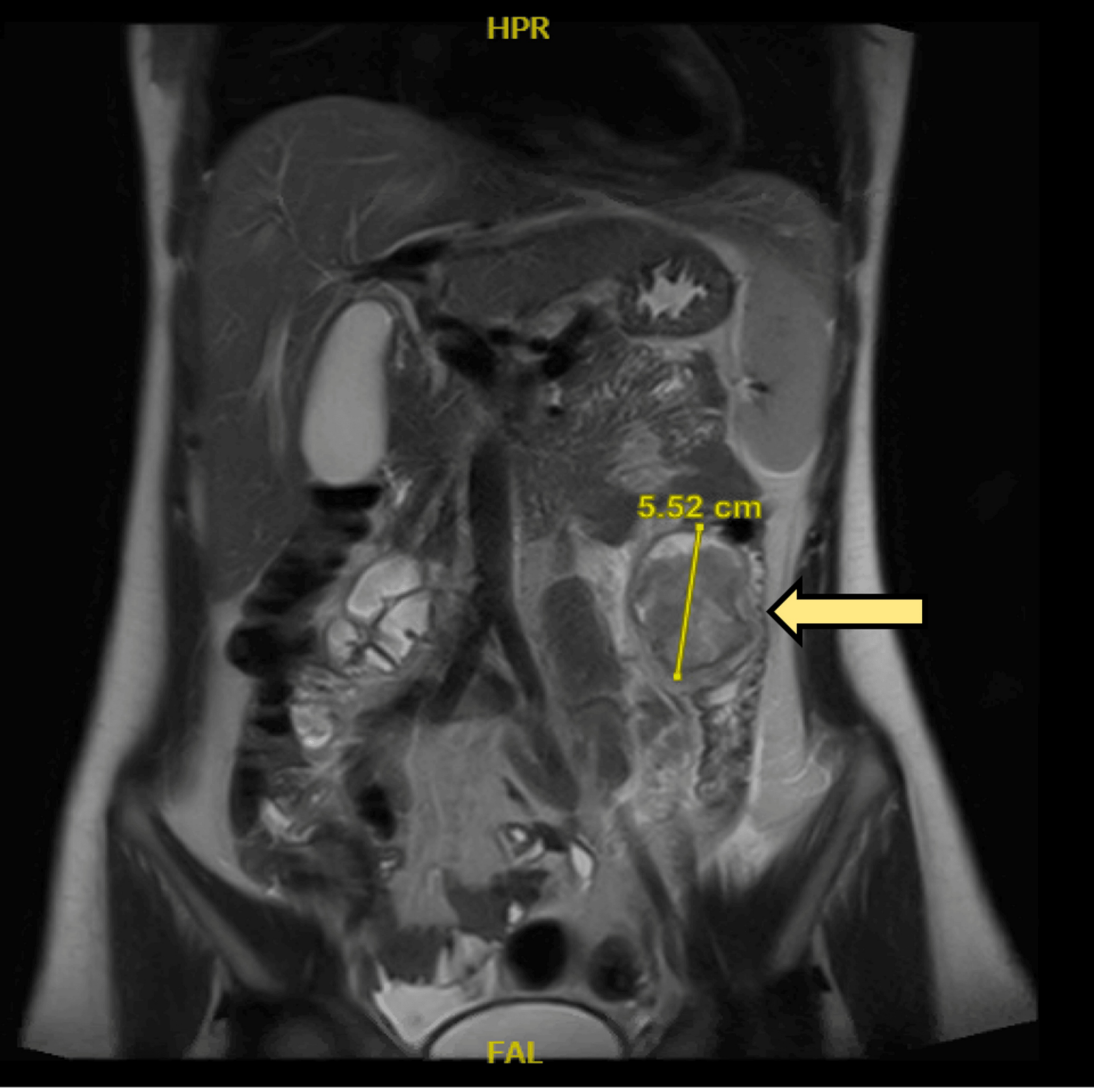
Axial view of magnetic resonance imaging showing left-sided colonic mass The yellow arrow is indicating the mass (the mass is measured 5.52 cm).
